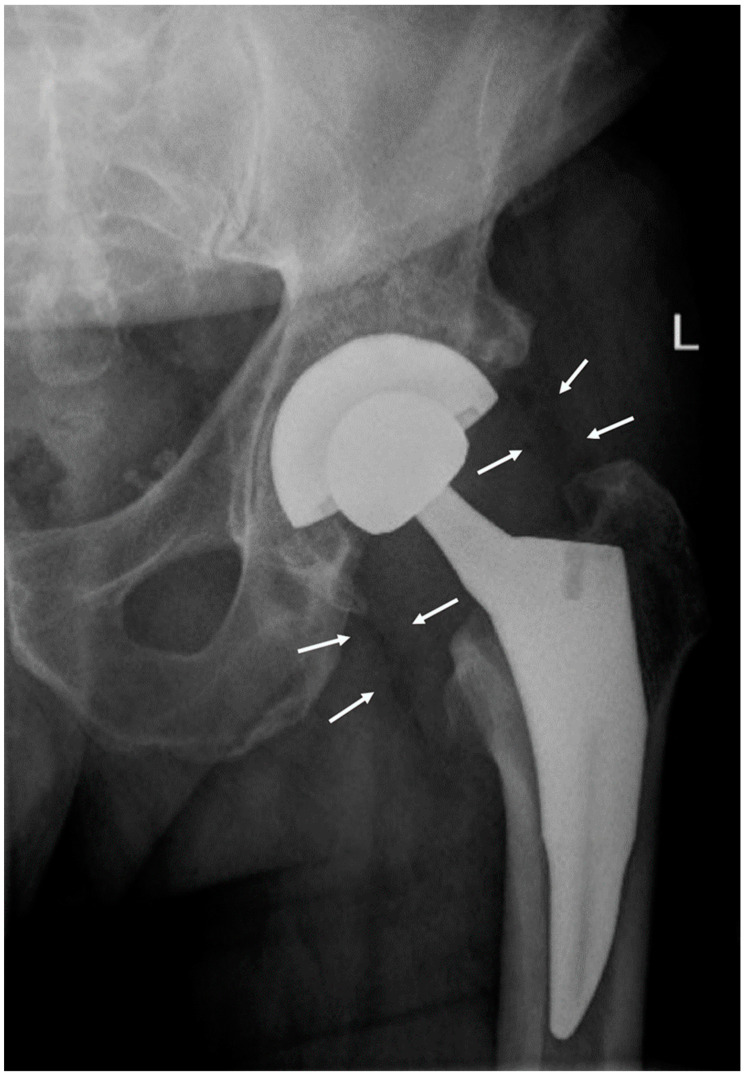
A hip radiograph demonstrating periprosthetic gas in soft tissue. Two foci of gas are outlined by white arrows; the largest focus of gas is at the lateral aspect of the hip. The patient underwent a total left hip replacement eight days prior to the onset of tetanus symptoms and demonstrated no other evidence of deep or superficial injury.
